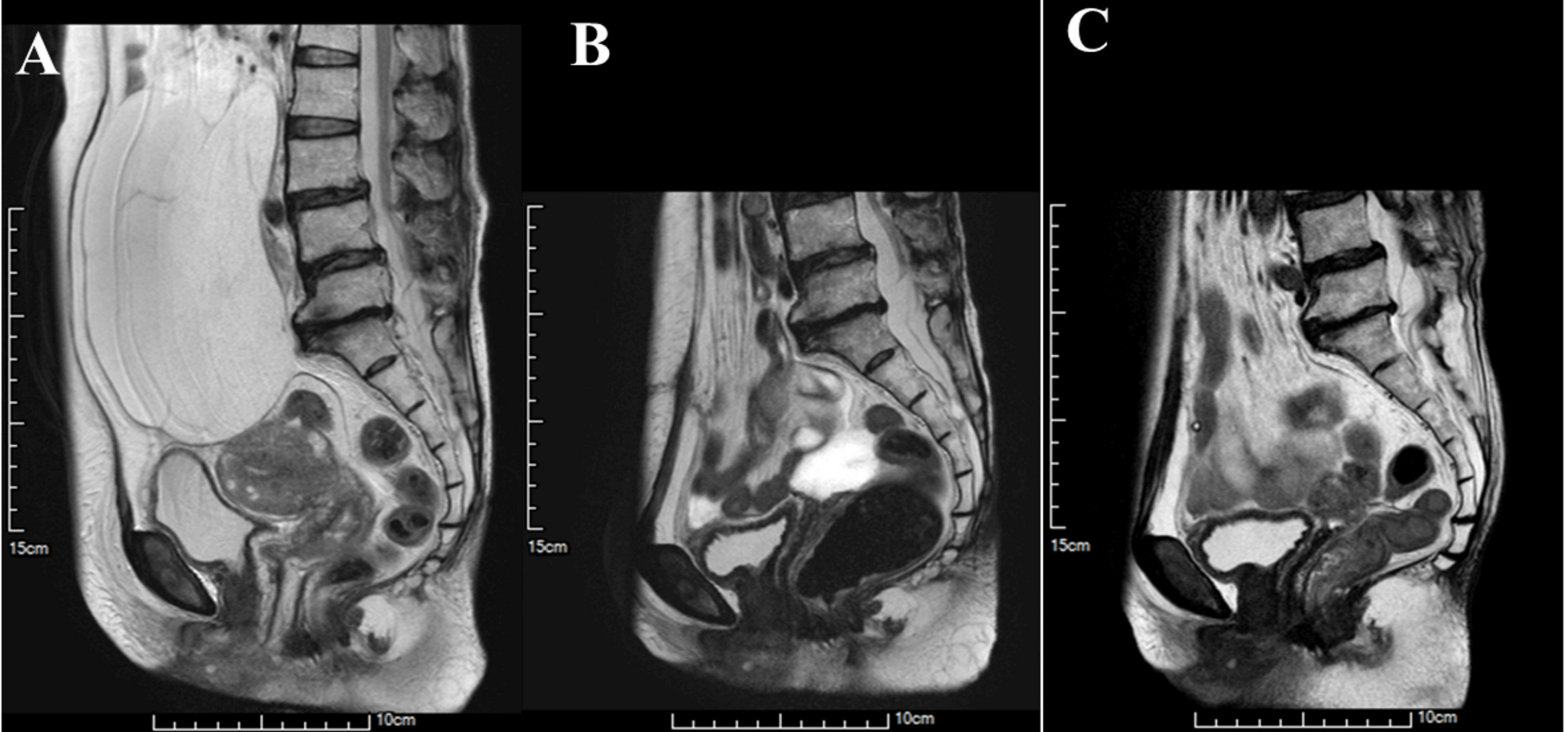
MRI images (A) before the first operation, (B) after the first operation, and (C) after the second operation. (A) Before the first operation, the MRI of the pelvis and abdomen shows a large multi-lobulated cystic mass measuring 20 cm in diameter, accompanied by somewhat viscous ascites; (B) After the first operation, the MRI reveals the presence of viscous ascites; (C) After the second operation, the MRI indicates no particular abnormalities.
